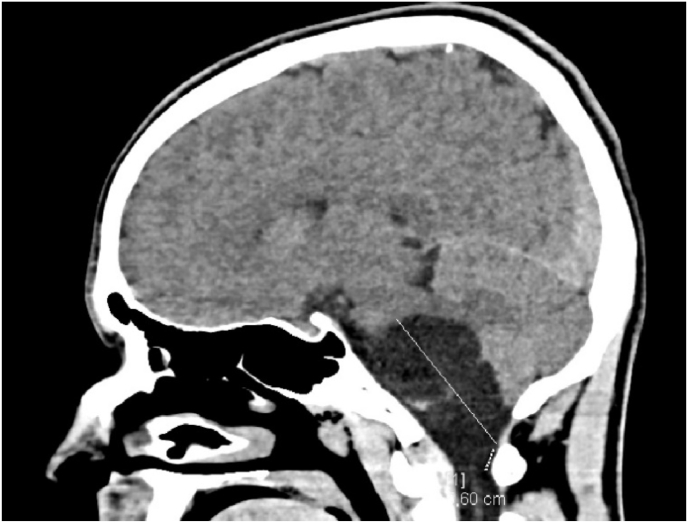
CT SCAN voluminous hypodense formation located on the left cerebellopontine angle.
